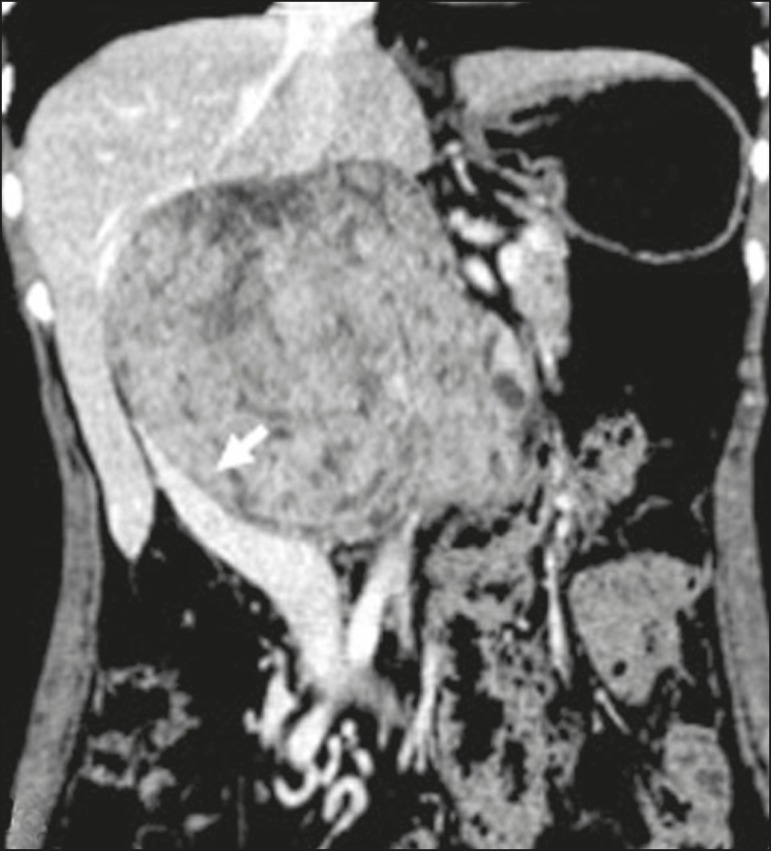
Retroperitoneal leiomyosarcoma in a 56-year-old female. CT scan
